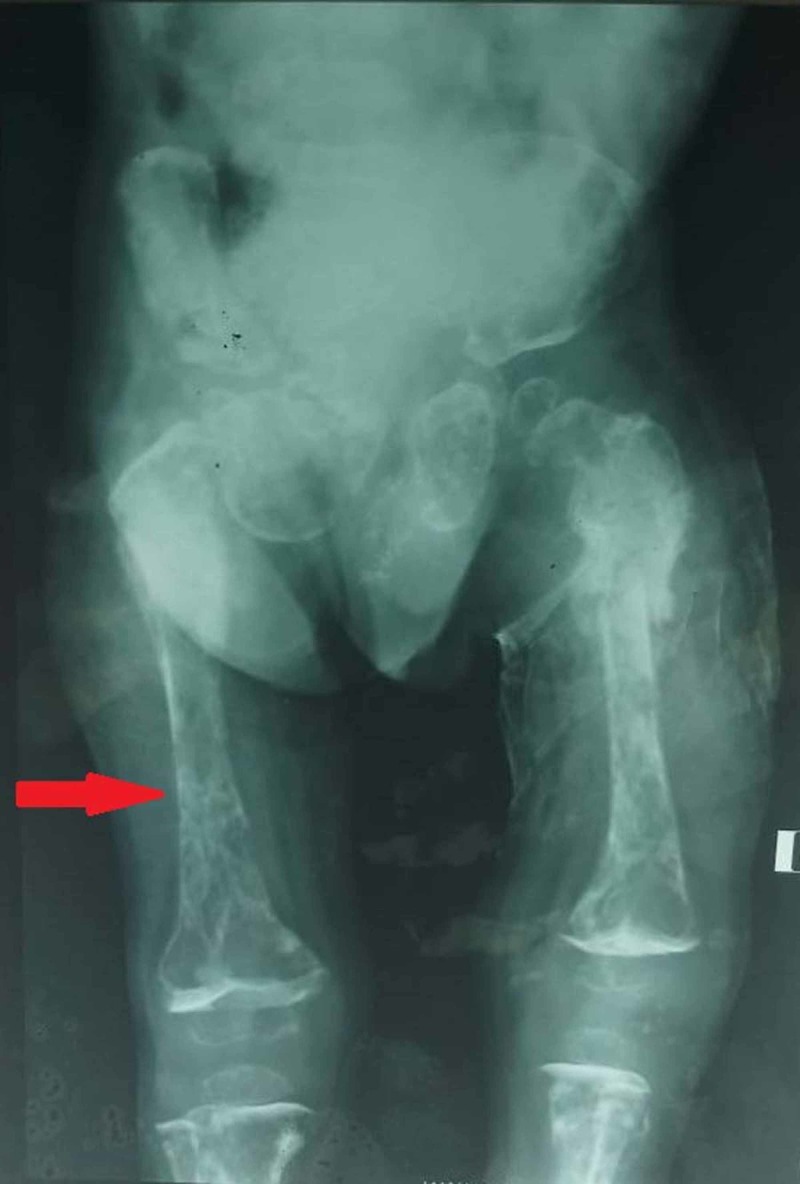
Femur X-ray of OI patient showing multiple femur fractures. OI: Osteogenesis imperfecta
